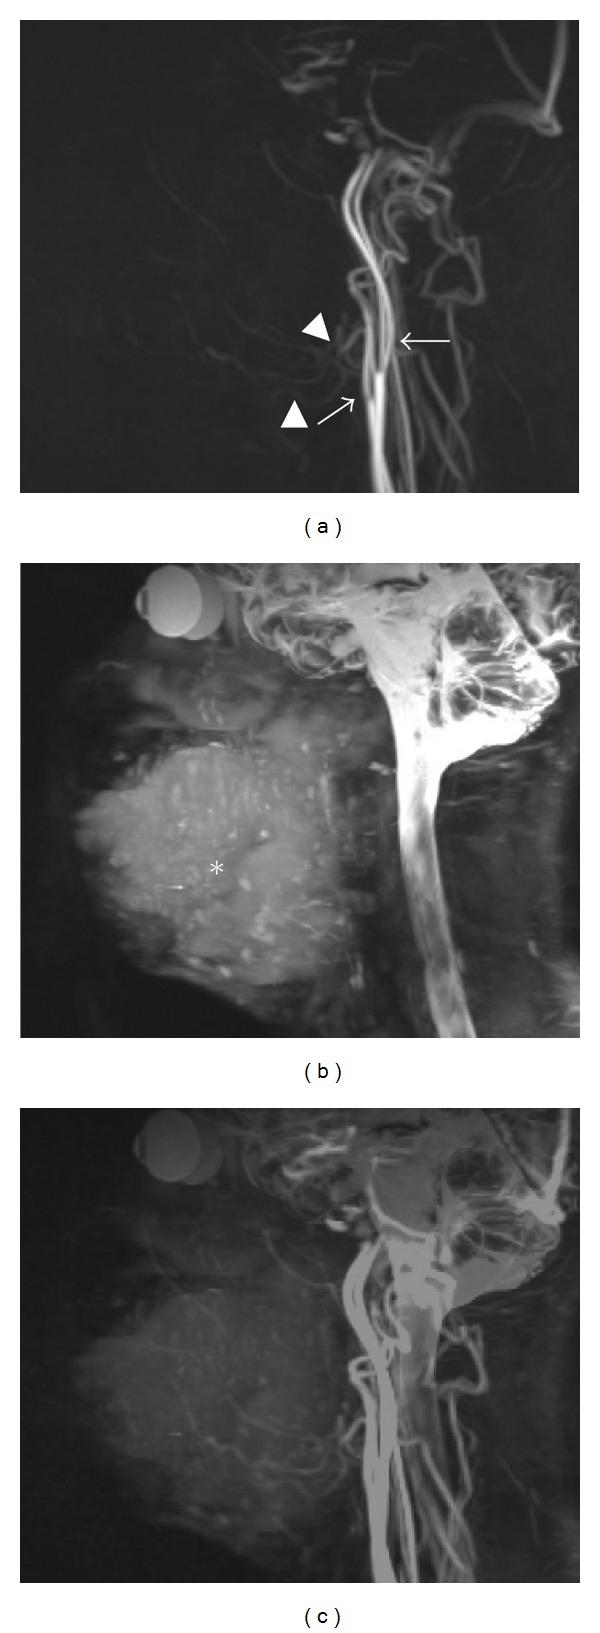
The production of a superimposed view of MRA using 3D-PC and a T2-weighted image in a 46-year-old patient with multiple hemangiomas of the bilateral tongue, lower lip, cheek, oral floor, masticator space, and right parotid space. (a) On MRA using 3D-PC, the external (arrow) and internal carotid arteries (black arrow), and the facial (arrowhead) and lingual arteries (black arrowheads) are shown, but the hemangiomas in the orofacial area are not seen. Acquisition time was 10 minutes and 15 seconds. (b) Fat-saturation T2-weighted image using 3D-FASE sequences of the head and neck. A hemangioma is visualized on this image (star). Acquisition time was 5 minutes and 8 seconds. (c) On fusing fat-saturation T2-weighted images with MRA by 3D-PC, hemangiomas and surrounding vessels can be visualized. However, image resolution of the fusion image is degraded compared to that of MRA using 3D-PC.
